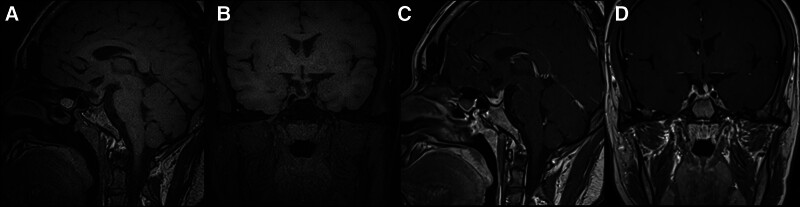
Patient MRI on October 22, 2023 (A and B:T1WI; C and D: enhanced MRI).
